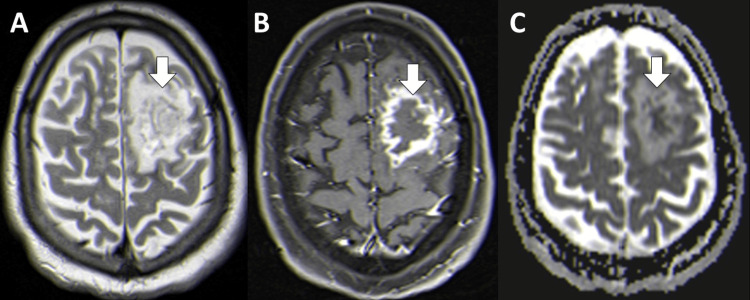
Brain magnetic resonance axial images evidencing a left frontal subcortical mass lesion (arrows) with heterogeneous high signal on T2-weighted imaging (A), a thin enhancing rim after contrast (B), and central restricted diffusion evident on the apparent diffusion coefficient map (C).
